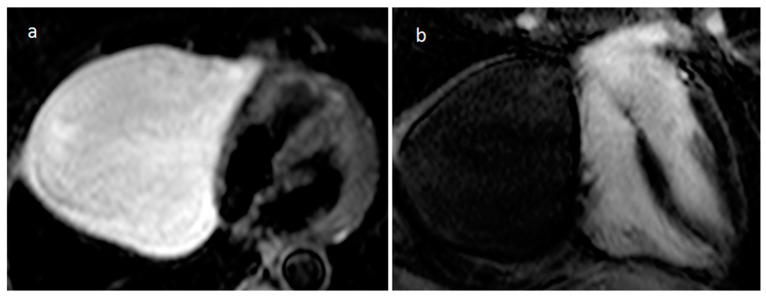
A 70-year-old female patient with a large right pericardiophrenic angle pericardial cyst on axial T2 STIR (a) and four-chamber view late gadolinium enhancement (b) MR imaging.
